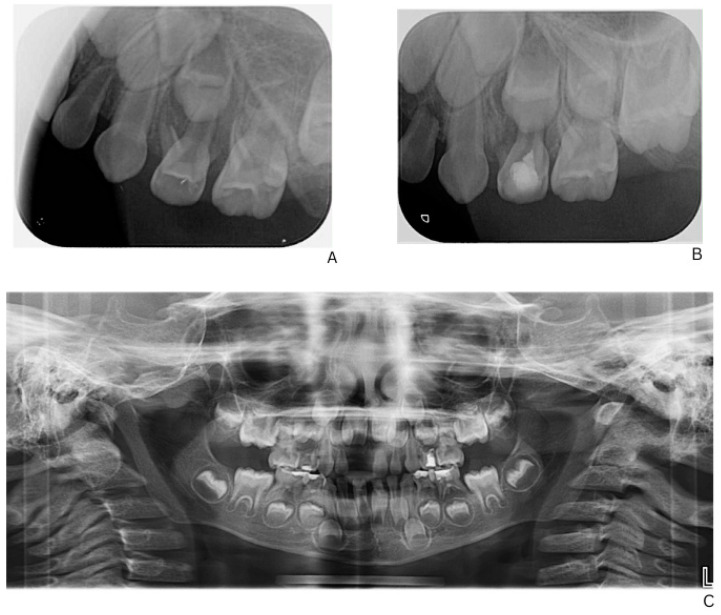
Radiographic follow-up of upper left first primary molar showing initial and post-treatment periapical status, and panoramic overview. (A) Pre-treatment periapical radiograph of the upper left first primary molar. (B) Post-treatment periapical radiograph showing healing after formalin–resorcinol endodontic protocol and glass–ionomer cement restoration. (C) Panoramic radiograph (OPG, 2025) demonstrating overall progression of dental treatment and status of other primary molars.
